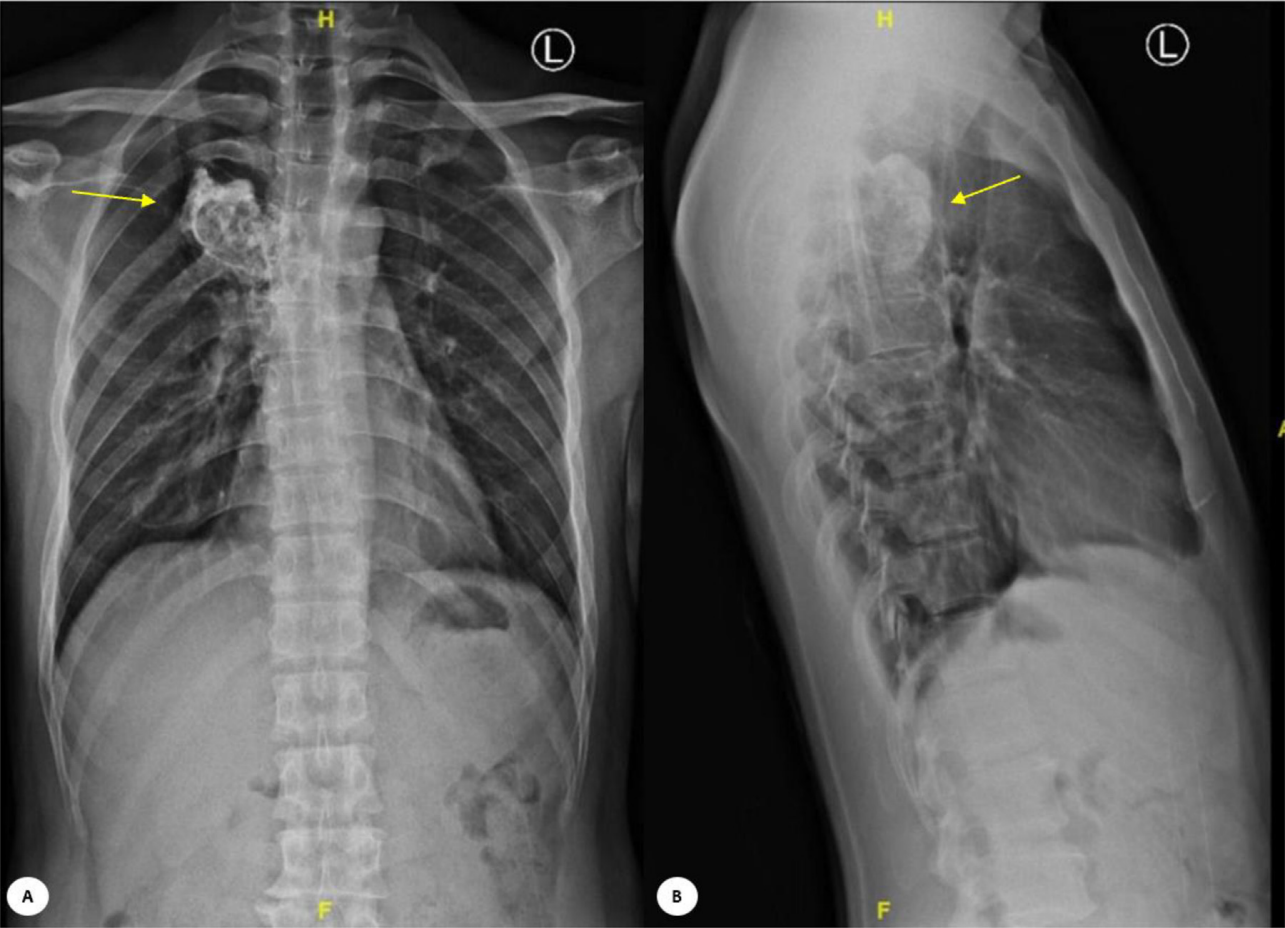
Conventional x-ray examination of the thoracolumbar spine (X- ray Carestream DRX Ascend 2018 with (A) Anterior-posterior and (B) Lateral view revealed a lytic sclerotic expansile lesion on the right posterolateral aspect of the thoracic vertebrae 5-6 (yellow arrow).
